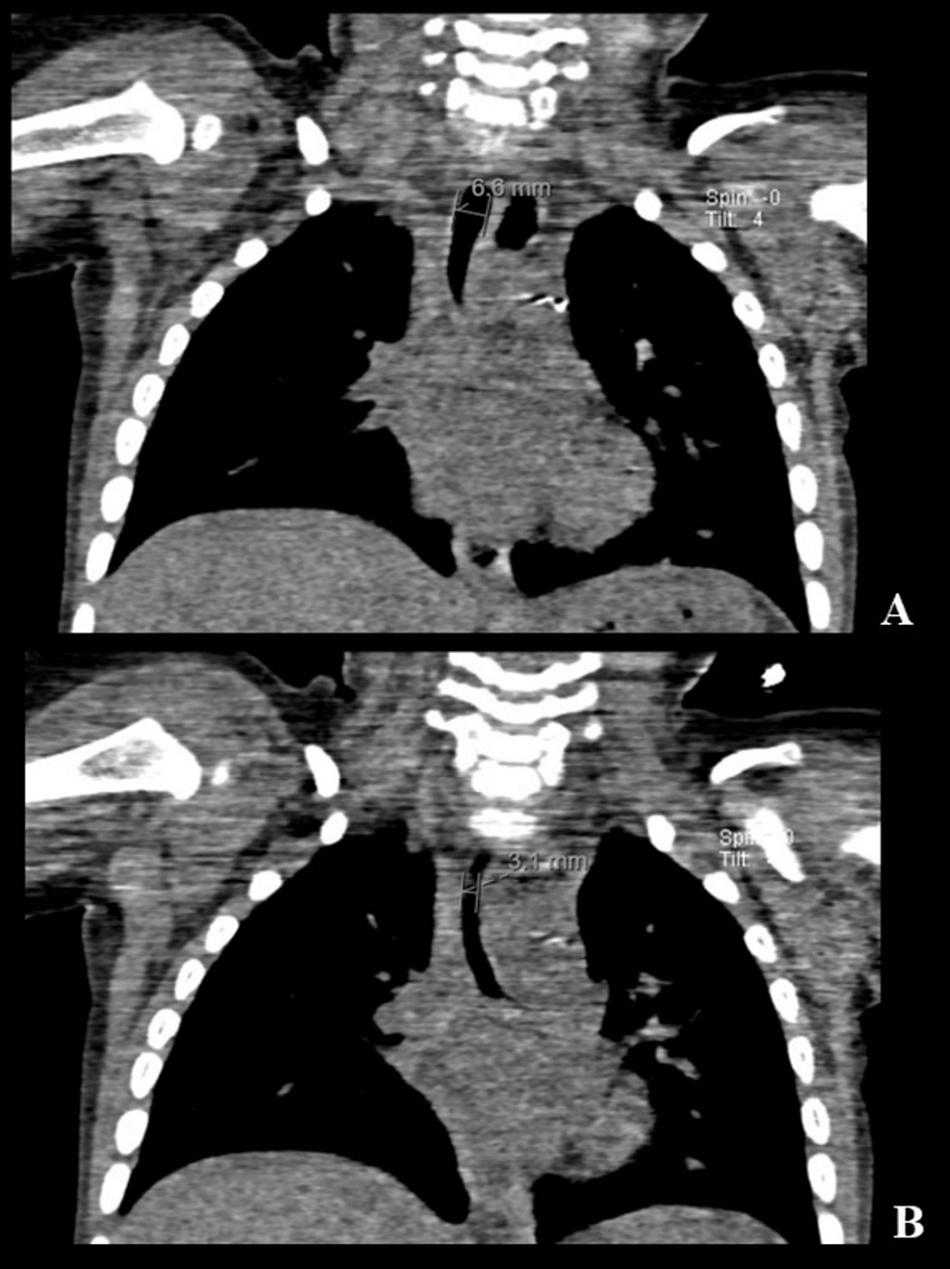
Computed tomography angiogram of the chest A: Coronal view showing a non-stenotic sub-cricoid trachea with a diameter of approximately 6.6 mm. B: Coronal view showing a stenotic distal segment of the trachea with a diameter of approximately 3.1 mm.
